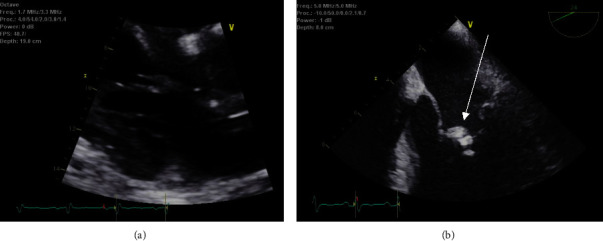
(a) Transthoracic echocardiography (TTE) and (b) follow-up transesophageal study (TEE) demonstrating a density (identified by arrow in (b)) on the mitral valve.
